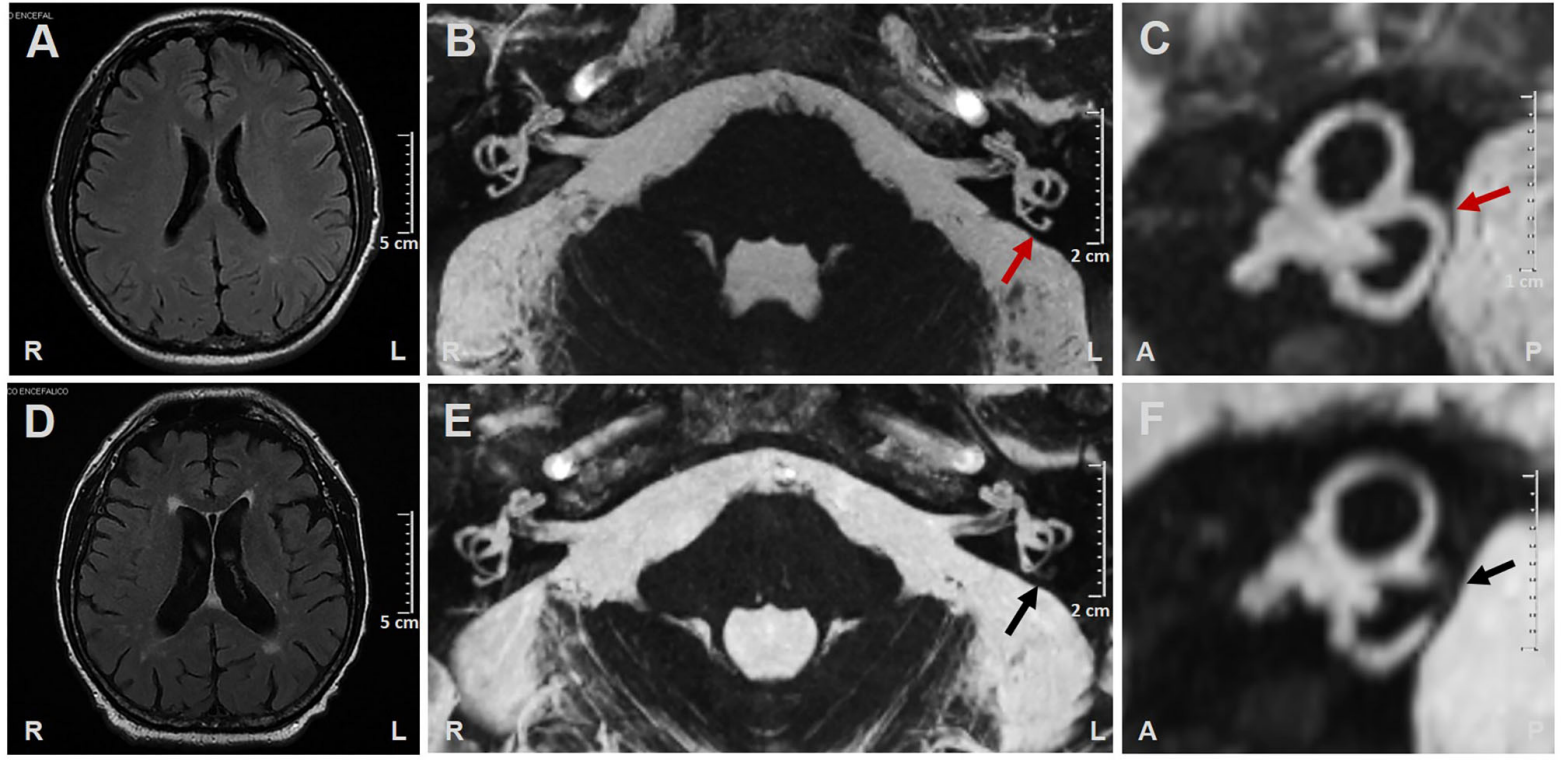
(A–C) Brain MRI performed 4 years prior to left-sided labyrinthine ischemia exhibiting (A) Slight signs of periventricular leukoaraiosis on axial T2-weighted FLAIR (fluid-attenuated inversion recovery) images. (B) Axial and (C) sagittal MRI with 3D-FIESTA (fast imaging employing steady-state acquisition) sequences showing normally fluid filled semicircular canals on both sides (red arrows indicate left posterior semicircular canal). (D–F) Brain MRI performed 14 days after left-sided labyrinthine ischemia showing (D) Increased areas of periventricular leukoaraiosis and widened cerebrospinal fluid spaces on atrophic basis on axial T2-weighted FLAIR images. (E) Axial and (F) sagittal MRI with 3D-FIESTA sequences showing filling defect within the left posterior semicircular canal (black arrows). A, anterior; L, left; P, posterior; R, right.
